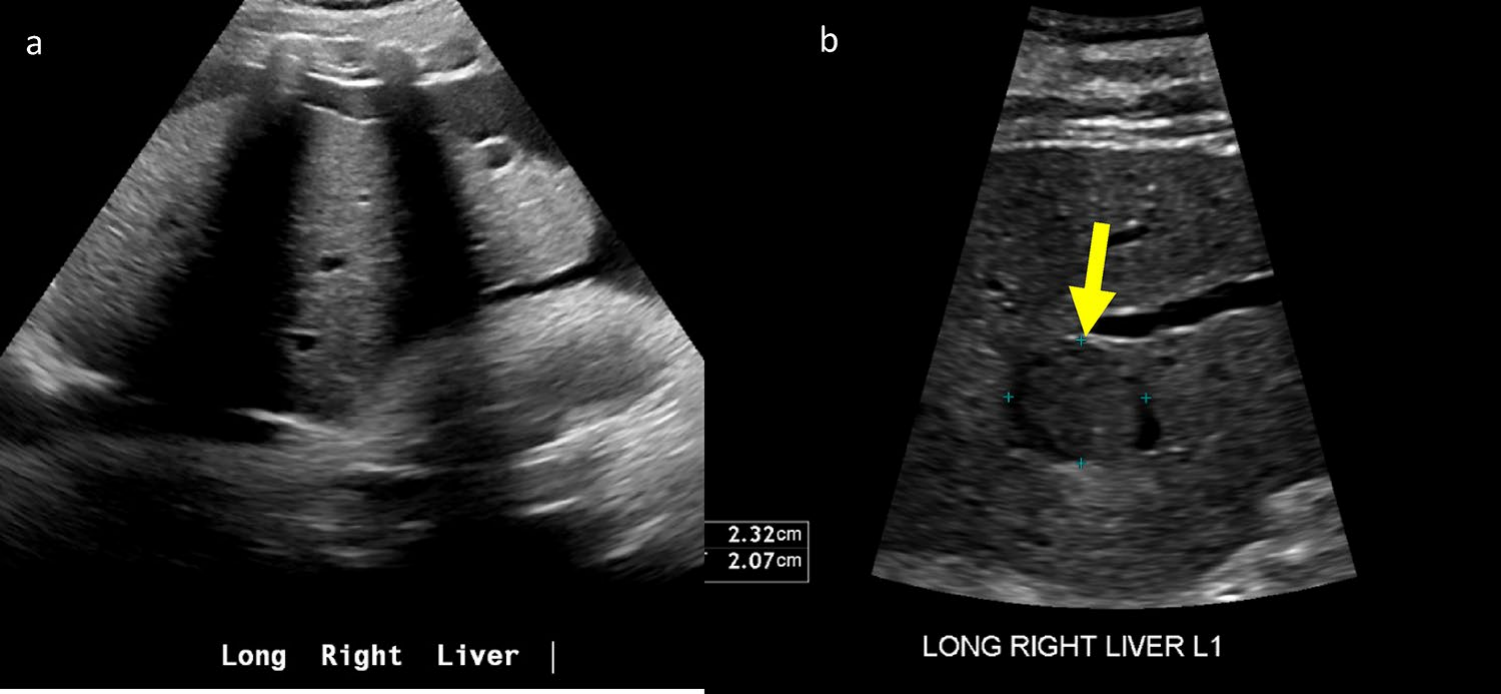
Visualization score improves in outpatient setting in a 60-year-old male with alcoholic cirrhosis. a Sagittal grayscale US image of the liver shows rib shadows and ascites, with no suspicious observation noted. The patient was hospitalized for decompensated cirrhosis and was unable to change positions or suspend respiration. b Sagittal grayscale US image of the liver performed a few months later in the outpatient setting shows a 2.3 cm hypoechoic solid nodule (arrow and in calipers), categorized as US-3 positive. This was characterized as LR-5 on subsequent MRI (not shown)
